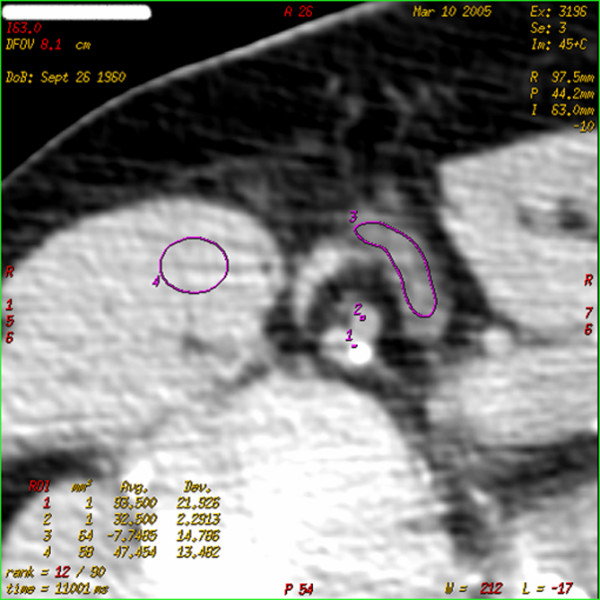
Image from one of the slices obtained by dynamic computed tomography centered on the palpable axillary node in patient AF with right breast carcinoma. A region of interest (ROI) was manually drawn along the margins of the target node (3). ROIs 1 and 2 were positioned in the axillary artery and vein. They were chosen with a very small area, as suggested by the software designer, to avoid partial volume effects. ROI 4 was drawn in the Teres major muscle for control.
